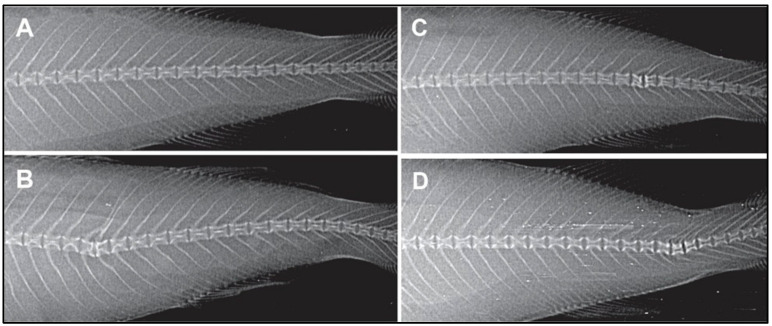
X-ray images of haddock (Melanogrammus aeglefinus) under different treatments: (A) control, (B) PAH exposure, and (C,D) oil exposure. The images show various vertebral malformations, including deformities in vertebrae. Reprinted from Meier et al. [57] under the terms of the Creative Commons Attribution License (CC BY). Copyright 2020, published by PLoS ONE.
